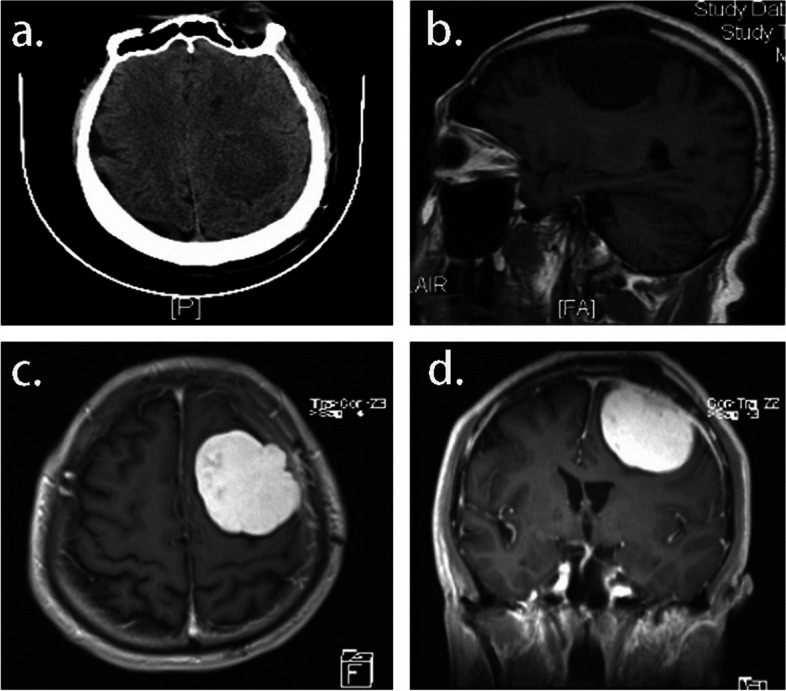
A Immediate preoperative CT performed at initial encounter demonstrated a minimally mass effect in the left parietal region. B Large well-defined mass lesion in the left posterior region with adjacent skull erosion was shown in sagittal T1. On axial (C) and coronal (D) enhanced T1, strong post-contrast enhancement was observed with dural tail sign, exerting mass effect on the left lateral ventricle with resultant shift of midline structures to the right
